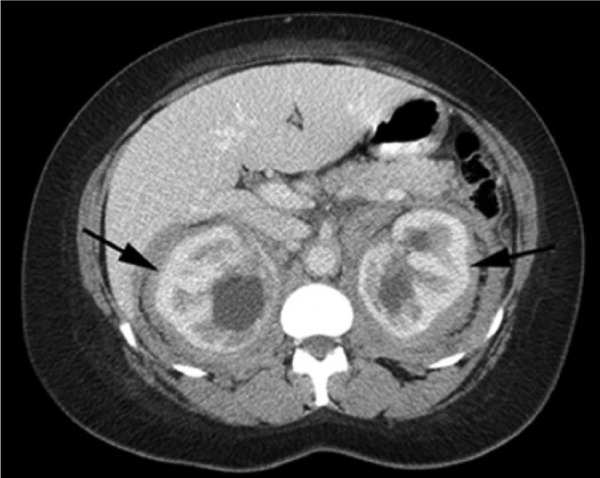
Radiologic findings. Computed tomography of the abdomen revealed soft tissue surrounding the aorta and kidneys, associated with inflammatory changes extending into the perirenal fascia bilaterally. Hydronephrosis was also present (arrows).
